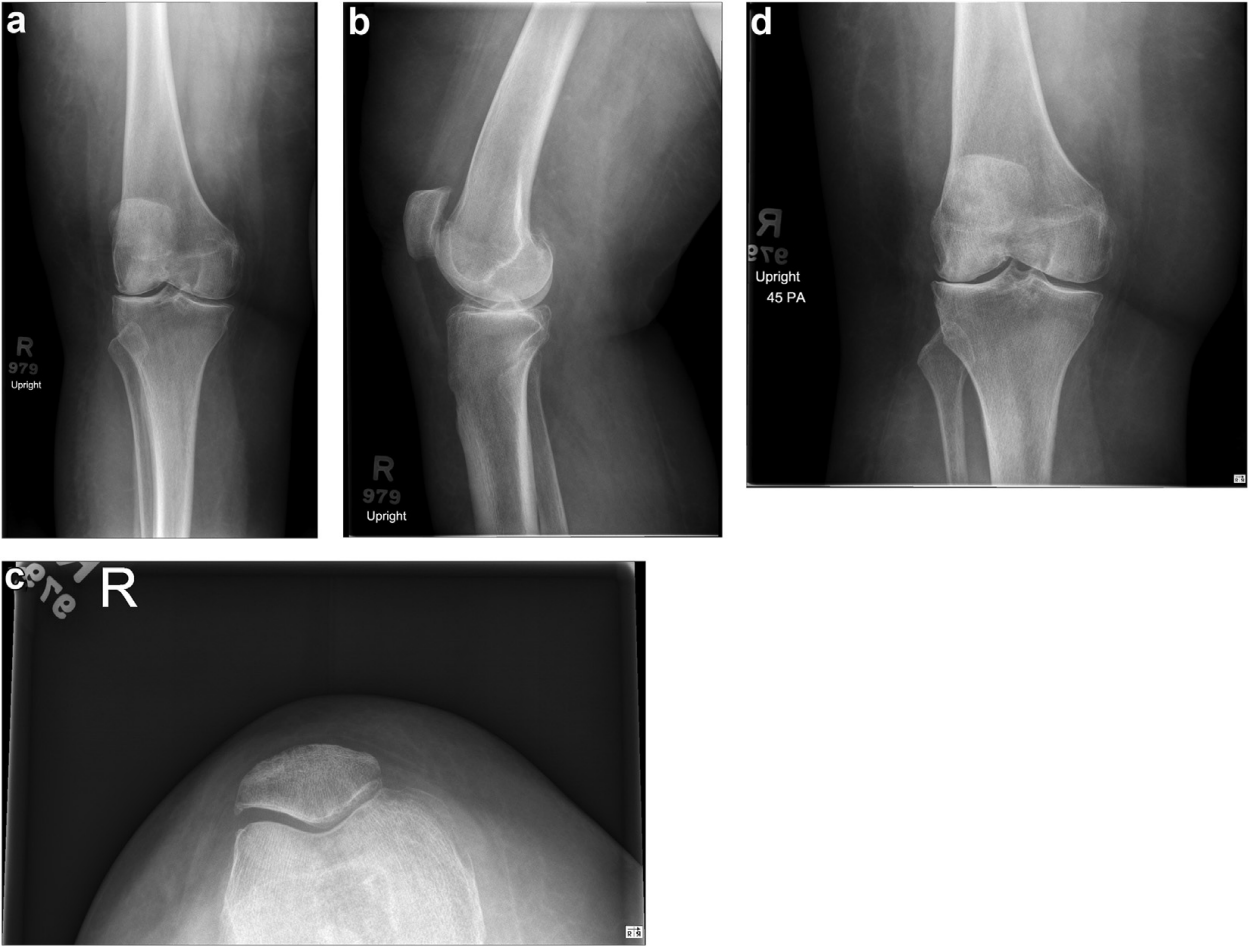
(a) Anteroposterior (AP), (b) lateral, (c) Merchant, and (d) Posteroanterior (PA) flexion knee radiographs demonstrate right tri-compartmental arthritis with valgus disposition.
